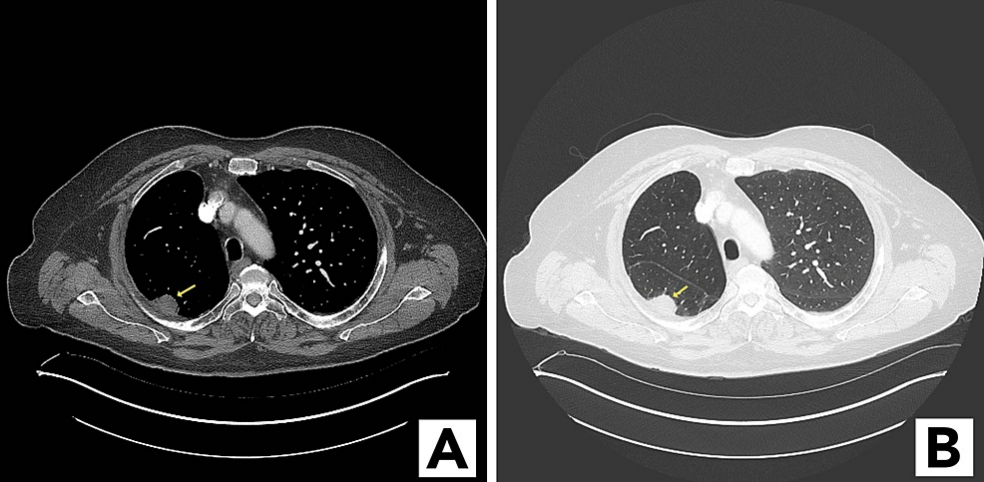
CT images showing nodular subpleural mass (arrow) in the right lower lobe. (A) Mediastinal window; (B) lung window
